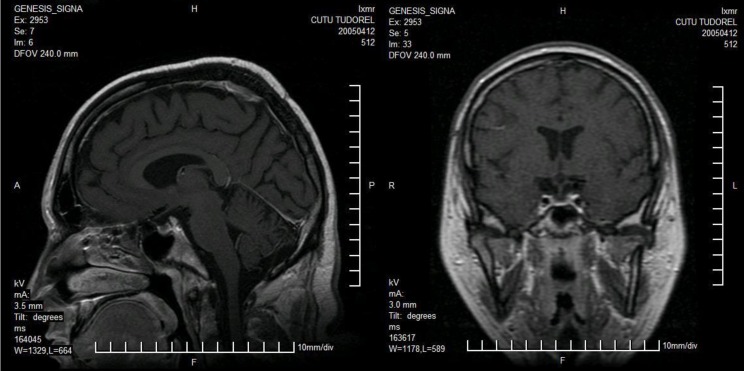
Magnetic resonance imaging – morphologic appearance suggesting “empty sella”
